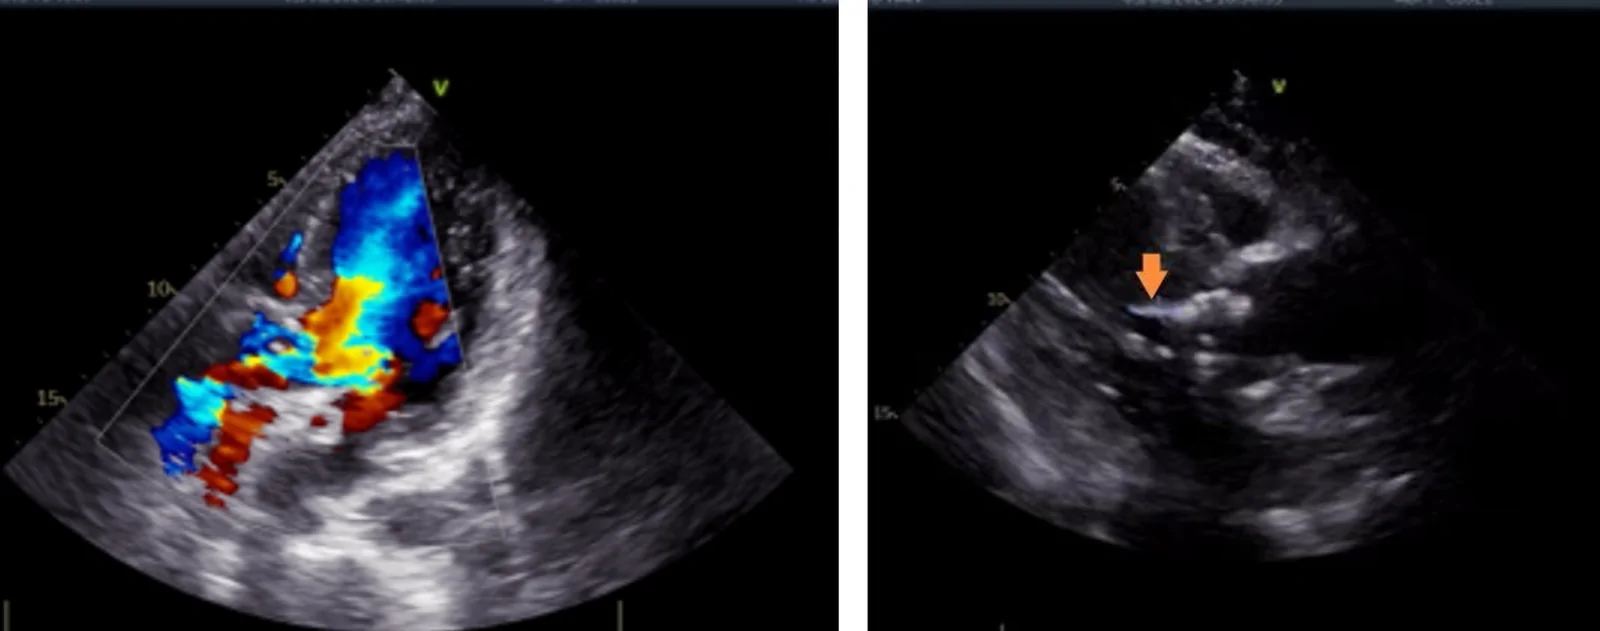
Echocardiography revealed massive aortic regurgitation with moderate stenosis and suspected vegetations. Yellow arrows represent suspicious vegetations.
